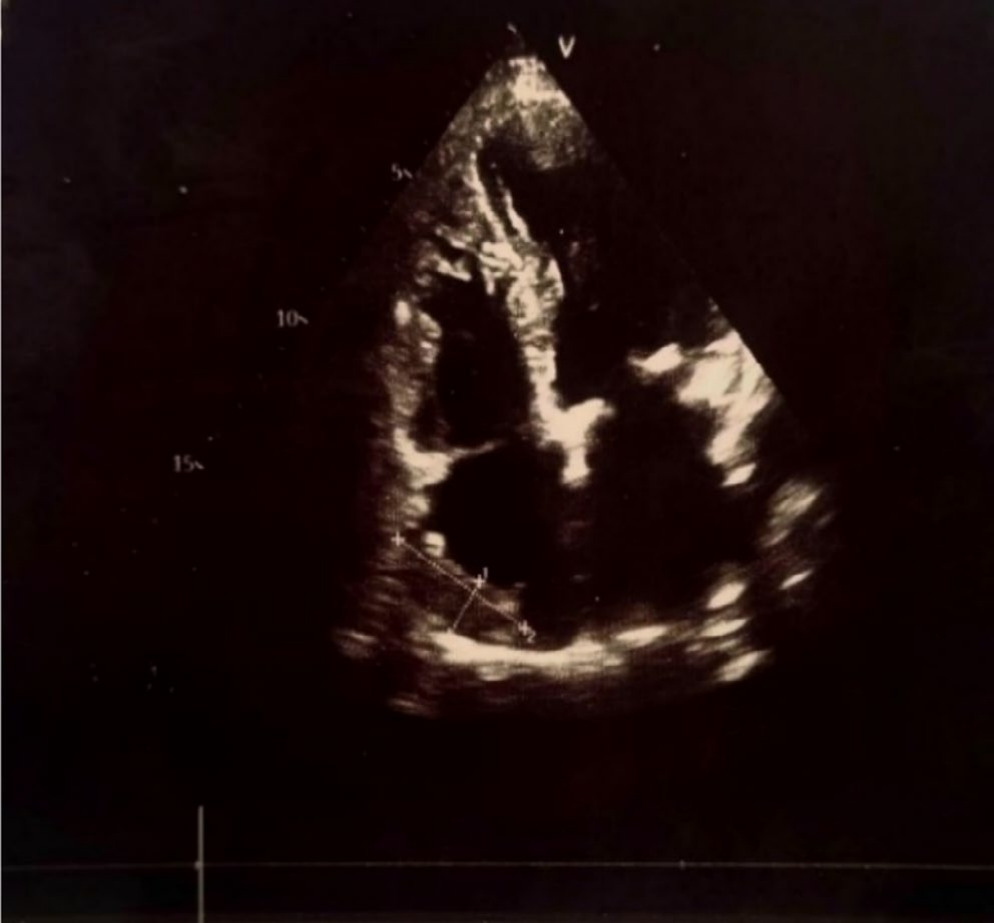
Transthoracic echocardiography showing a large intracardiac mass located in the right atrium.
